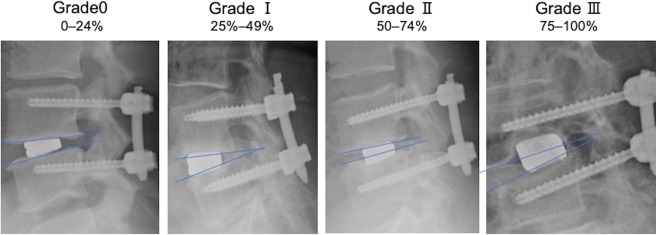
Subsidence grading. Radiographs showing examples of the different subsidence grades (0-III).
